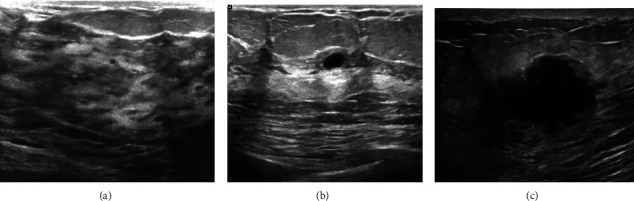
Three types of images in the dataset of breast ultrasound images. (a) Norm. (b) Benign. (c) Malignant.
